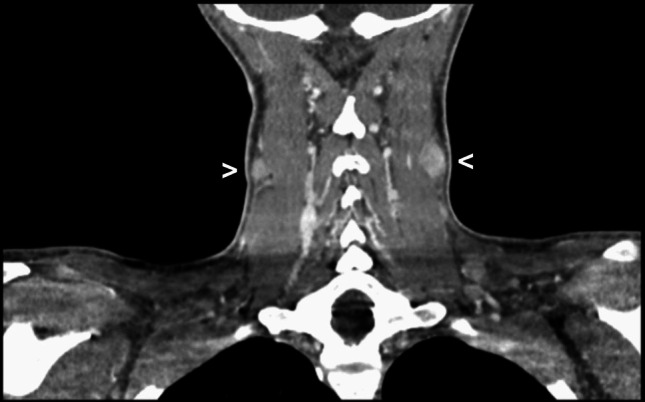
Radiologic presentation of cervical lymphadenopathy. Computed tomography scan of the neck showing bilateral cervical lymphadenopathy (arrowheads)
